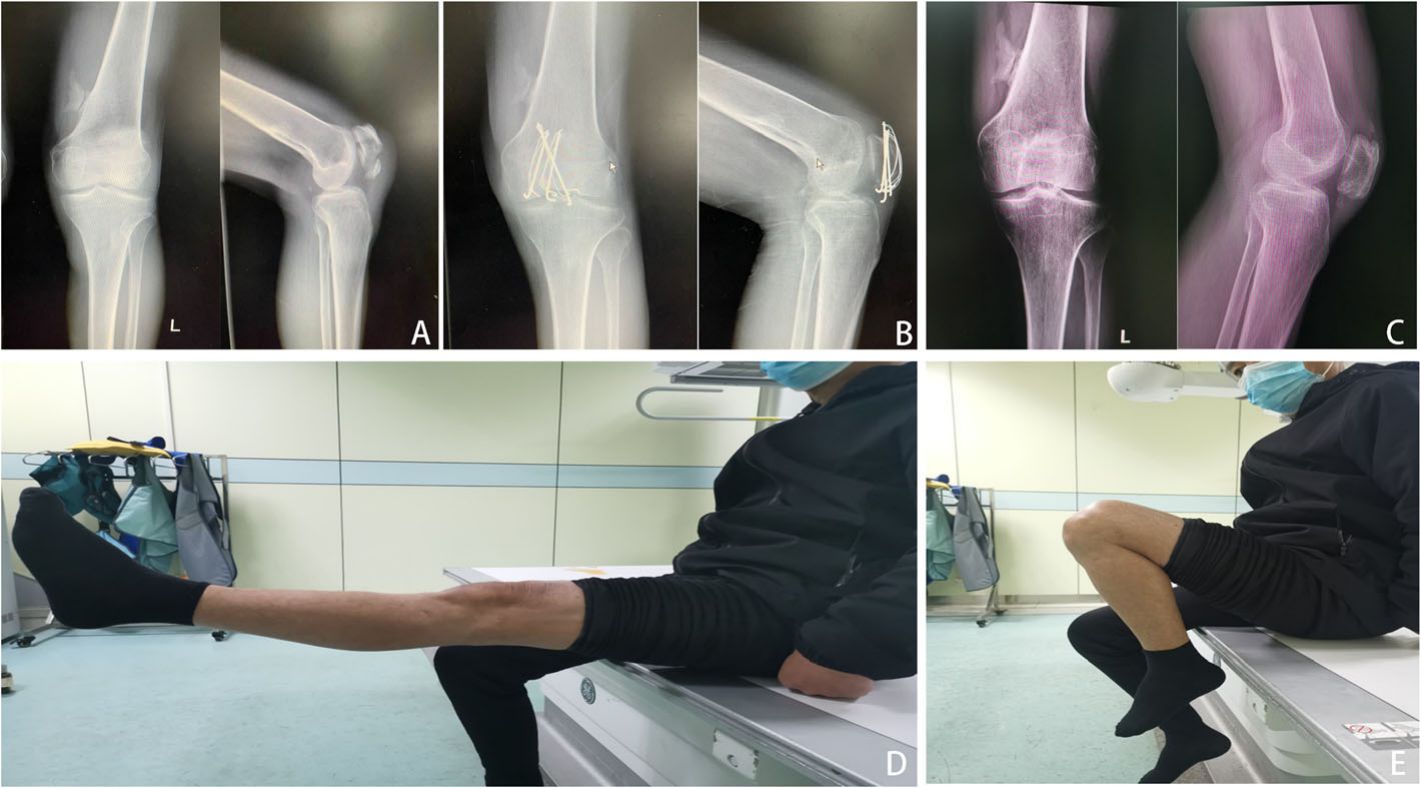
A Male, 58 years old, traffic accident, anteroposterior and lateral radiographs of the left knee showed patellar comminuted fractures preoperatively and B postoperatively at 1-year showed fracture healing. C Anteroposterior and lateral radiographs of the left knee postoperatively at the final follow-up showed patellofemoral arthritis. D Range of motion was restored normally at the final follow-up
